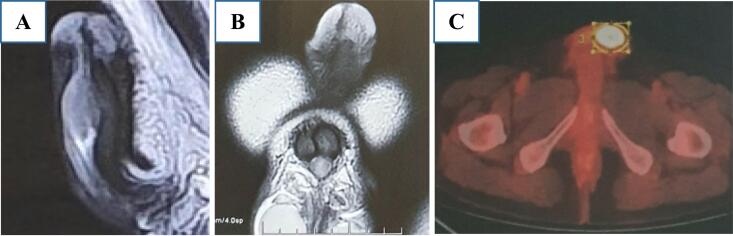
Shows MRI scans (A) and (B) indicating that cancer has invaded the left corpus cavernosum and occupies nearly the entire urethra, (C) A malignant hypermetabolic tumor is observed on the tip of the penis on PET/CT.
